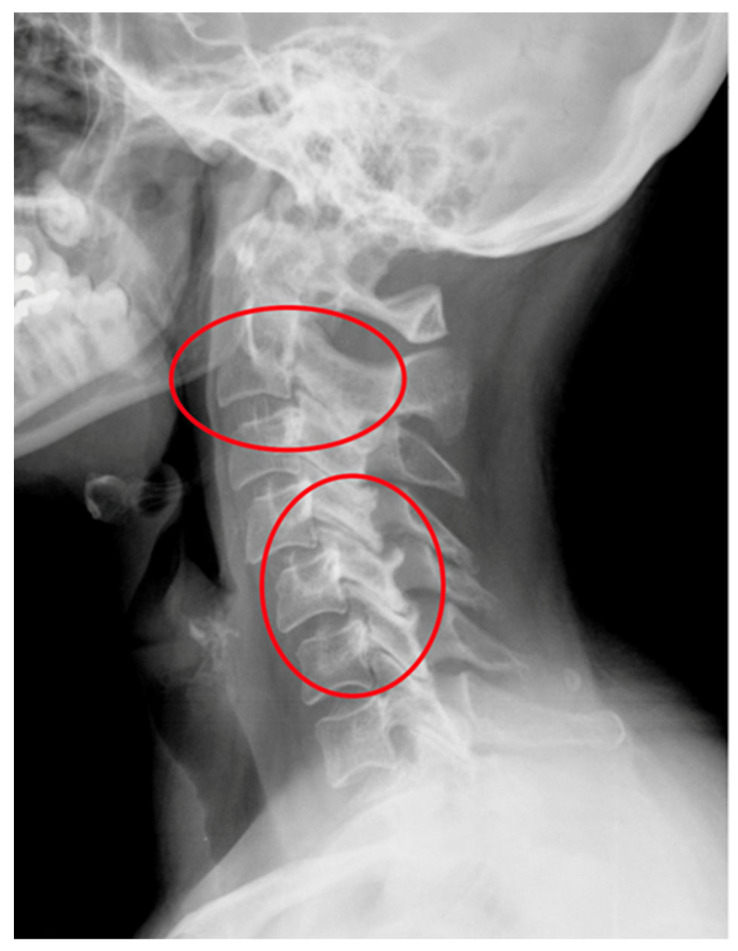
An X-ray of the cervical spine (lateral view) of a 55-year-old female patient with RA and SAS instability.
